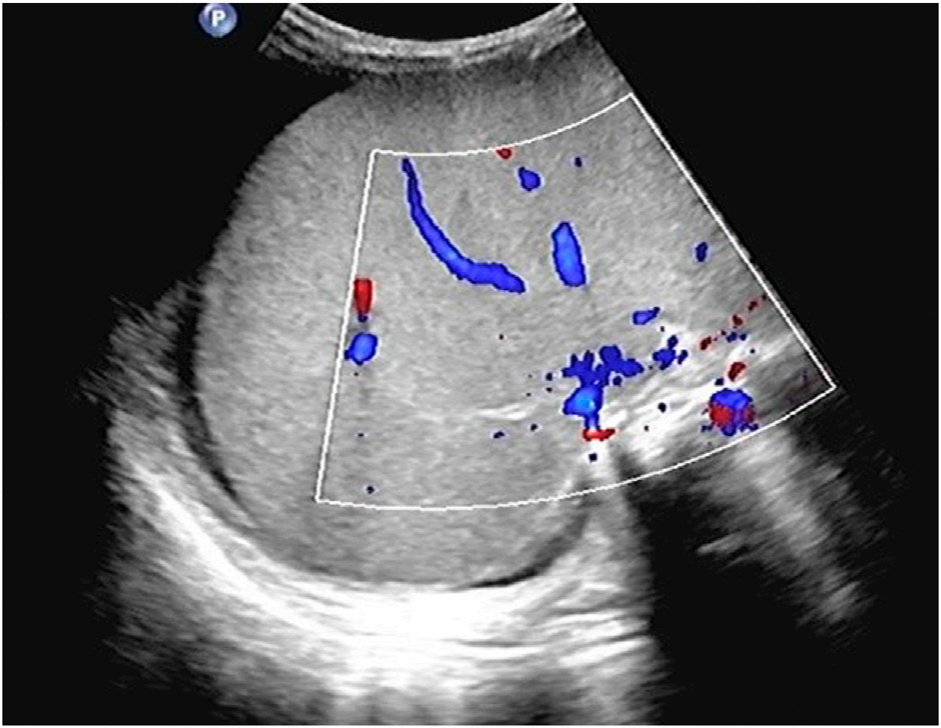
Results of abdominal ultrasound examination suggest ascites and right hepatic vein occlusion. There was an anechoic area around the liver, and the right hepatic vein lumen was not clearly displayed, and there was no obvious blood flow signal in the right hepatic vein course area.
